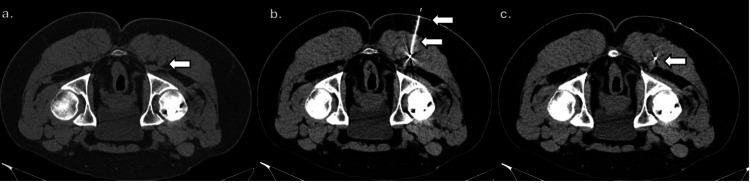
CT-guided preoperative localization with coil placement CT-guided localization of the deep solid tumor within the gluteal musculature (white arrow), isodense to muscle, performed with the patient in the prone position (a); a needle was advanced toward the tumor (double white arrows) (b); and under CT guidance, a platinum coil (white arrow) was deployed along the posterior margin of the lesion (c).
